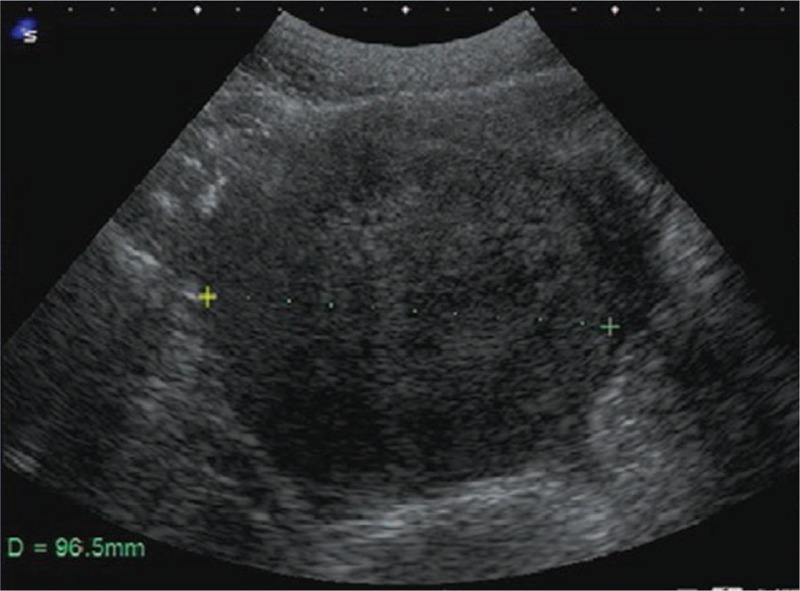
Transabdominal ultrasonographic image. There was a solid mass measuring 9.6 cm behind the uterus. The tubes and ovaries were not visualized because they were obscured by the enlarged uterus.
